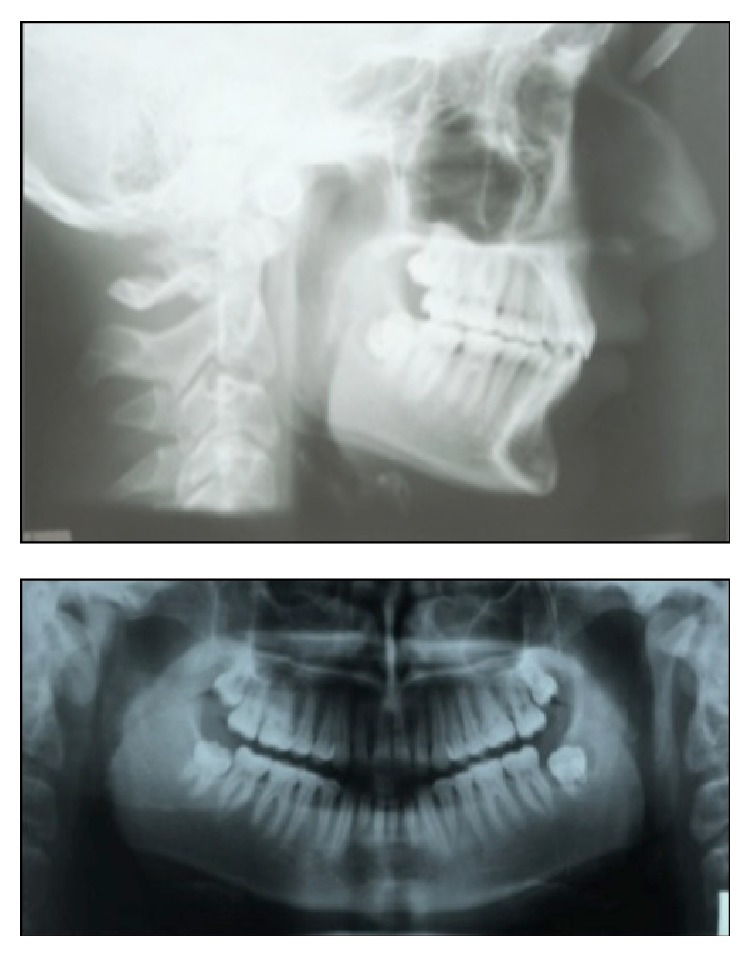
Posttreatment lateral and panoramic radiographs.
